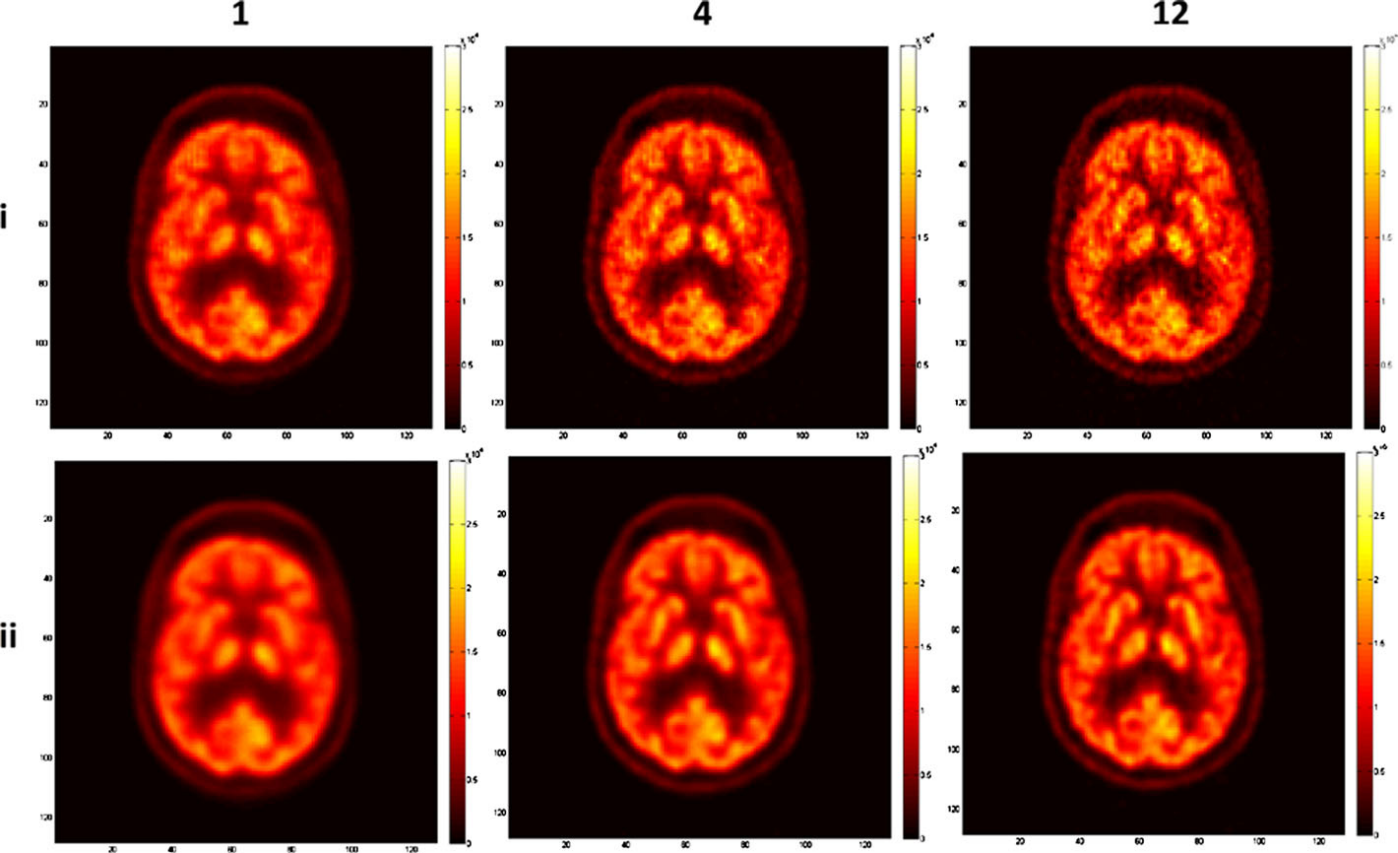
Reconstructed images of a clinical FDG-PET brain study using from left to right 1, 4, and 12 iterations with two parameter combinations: alpha = 1.3084, *r* = 1.6302, *d* = 2.0375 (*i*) and alpha = 10.4101, *r* = 3.9451, *d* = 2.0375 (*ii*).
